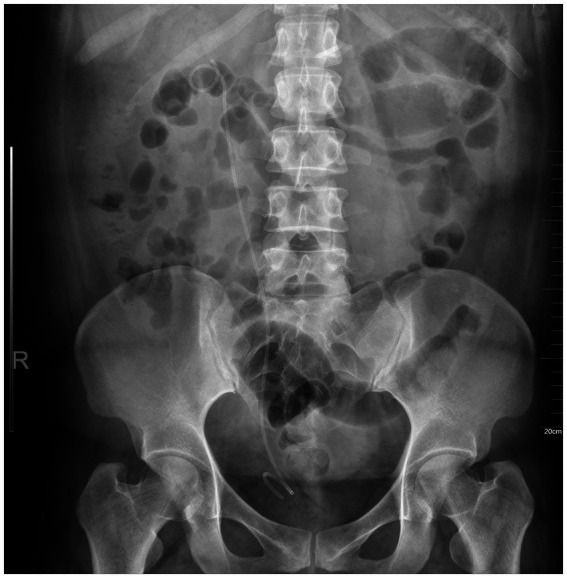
Postoperative plain abdominal radiograph demonstrating optimal positioning of the right double-J ureteral stent.
